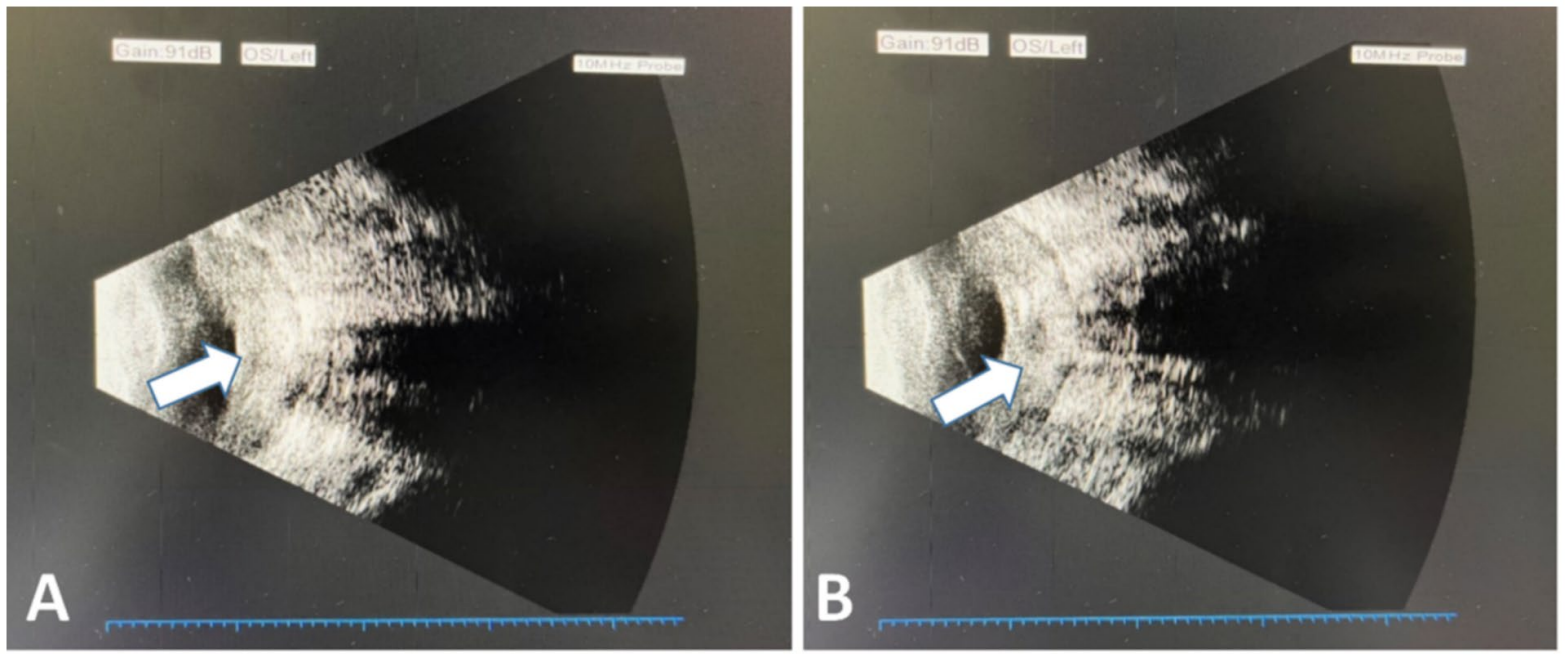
(A, B) B-scan ultrasonography demonstrating extensive vitreous opacities (white arrow).
